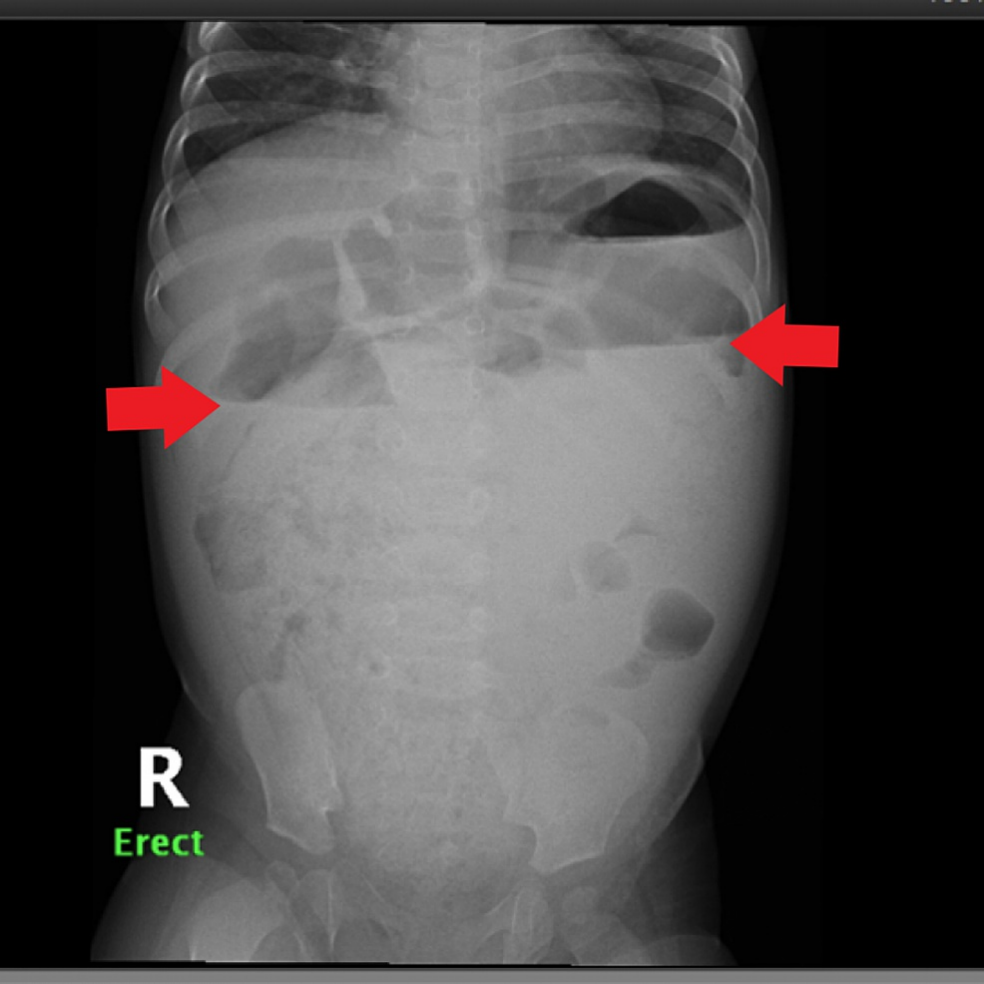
Abdominal erect X-ray showed a distended large colon by air and fecal matter with two air-fluid levels at the ascending and descending parts of the large colon
